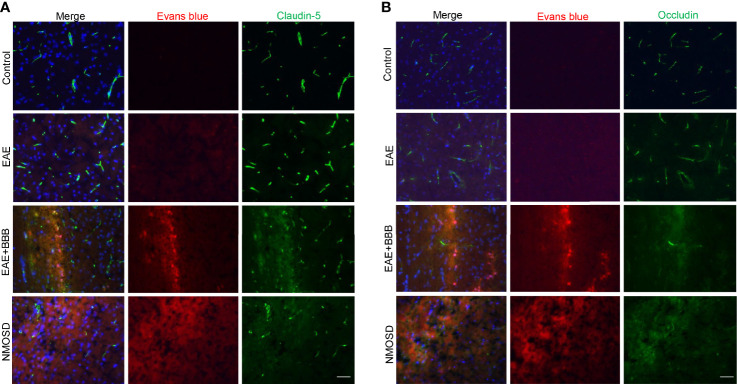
Loss of tight junction components following low-frequency ultrasound. (A, B) Immunofluorescence revealed loss of the tight junction protein, occludin and claudin-5, following low-frequency ultrasound.
